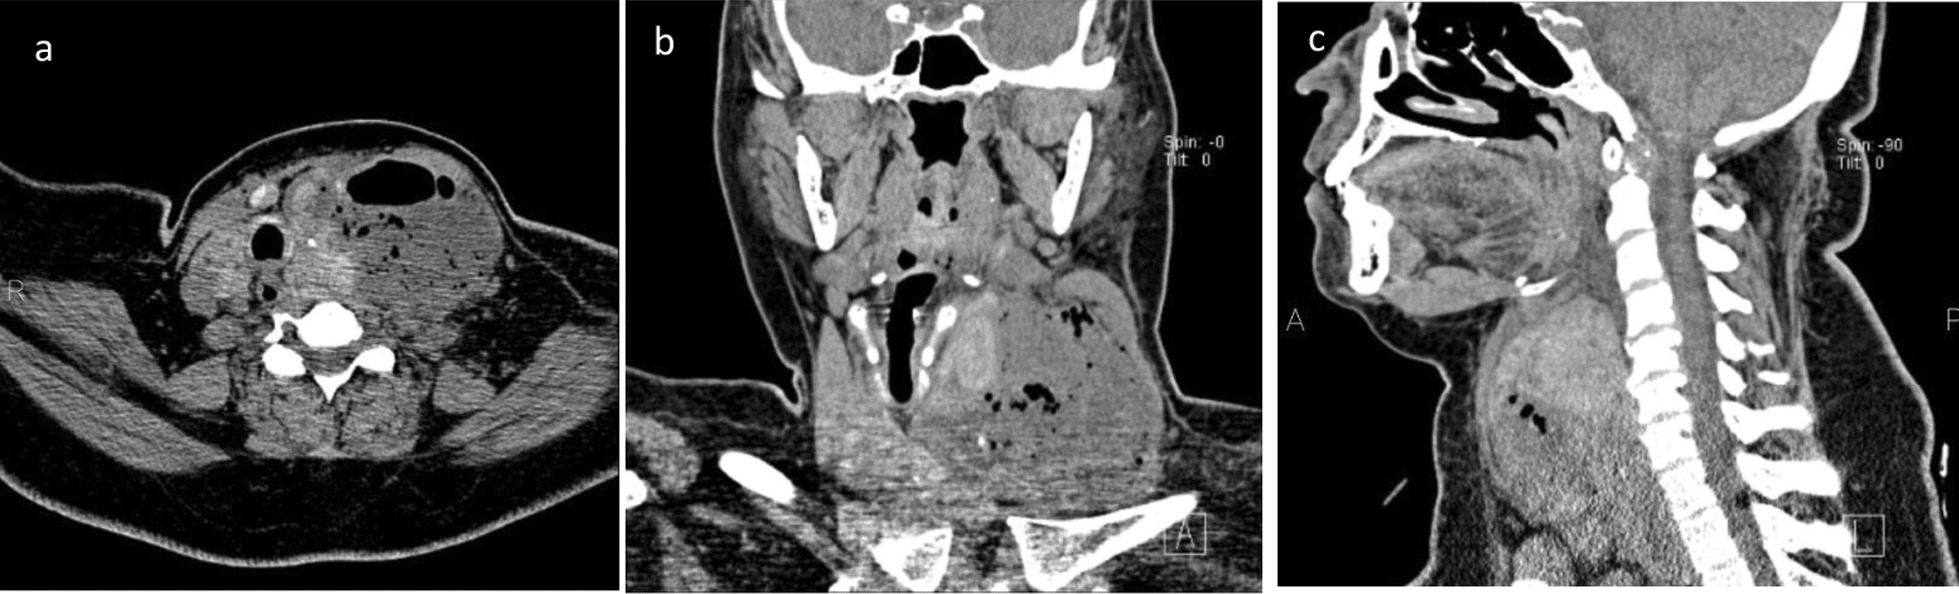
Axial, coronal, and sagittal (a, b, c) computed tomography images showing a well-defined gas-filled collection with enhancing margins in the left thyroid lobe plus widespread edema surrounding the left thyroid lobe; the trachea was shifted to the right side of the neck
